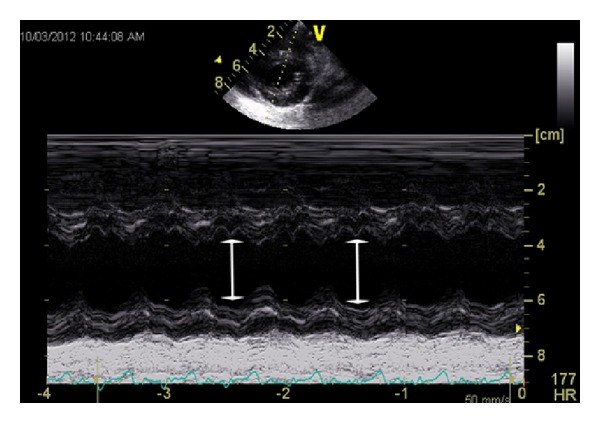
M-mode echocardiographic image, at 1-year follow-up, showing asynchronous motion of the interventricular septum during diastole, marked by arrows.
